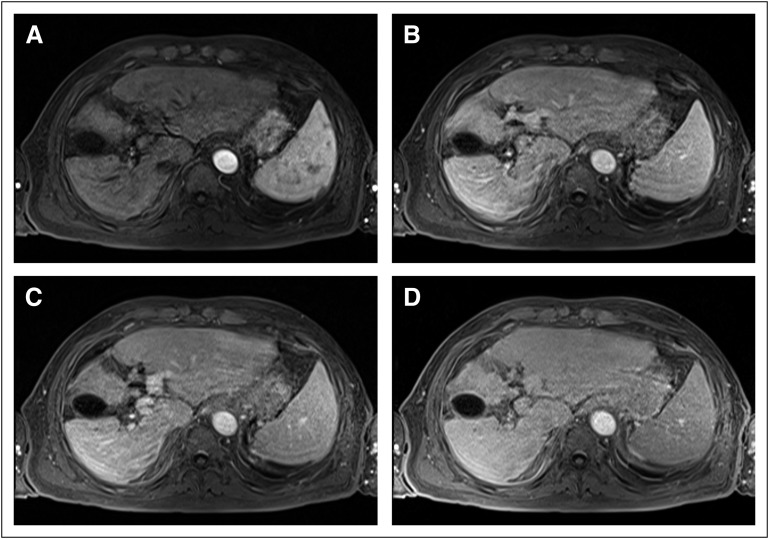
Axial sequence volumetric interpolated breath-hold examination T1-weighted
